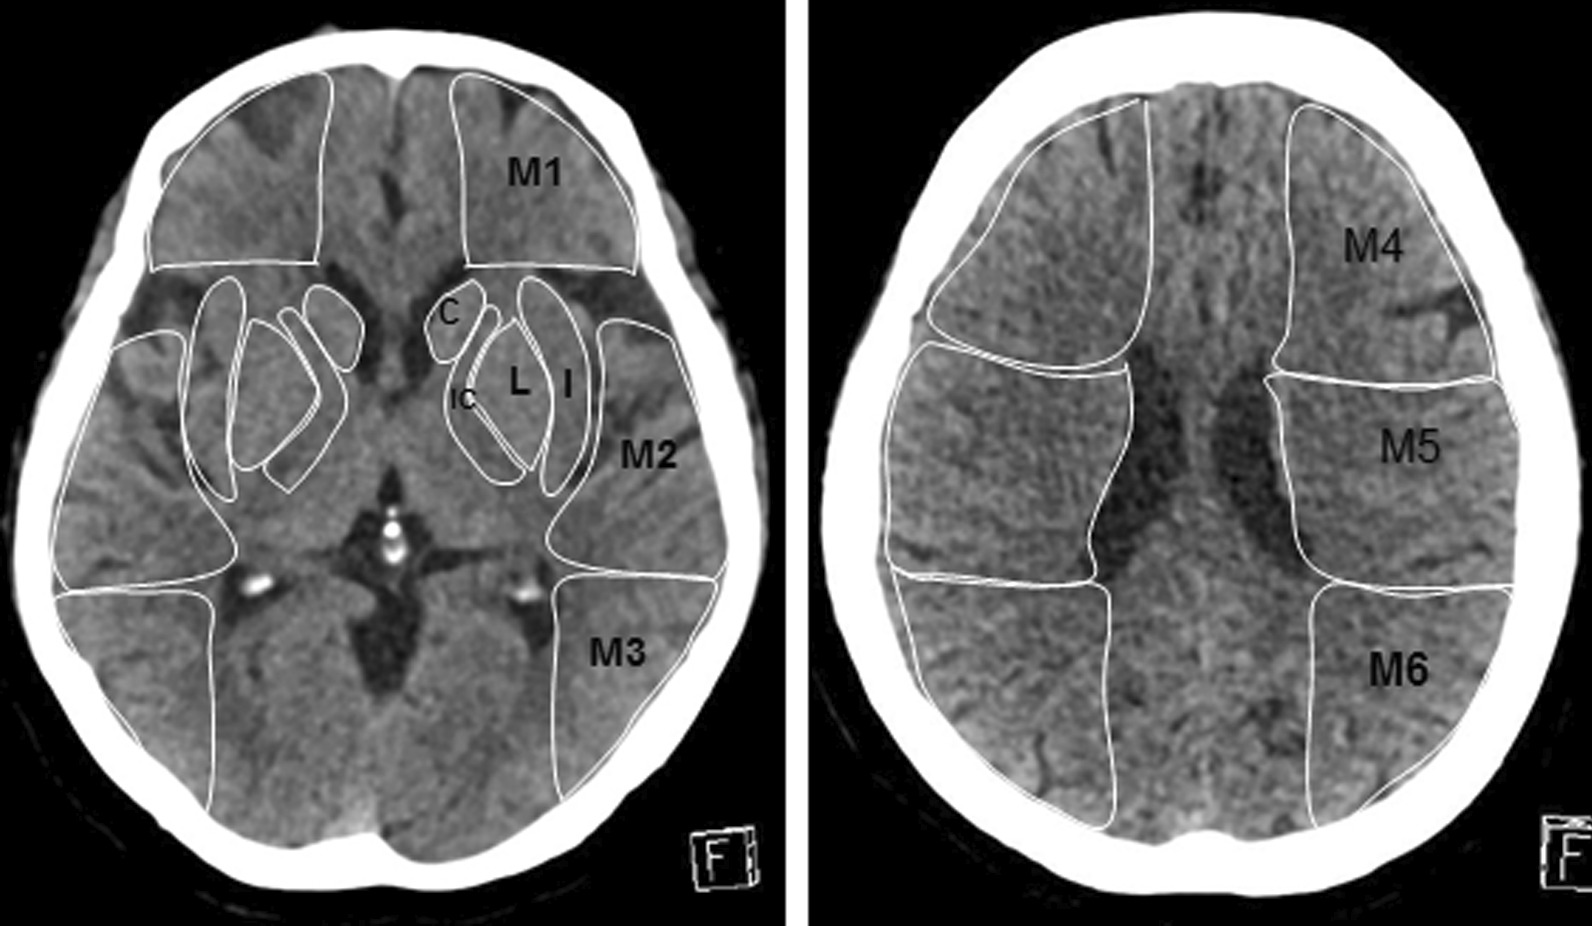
Ten ASPECTS regions were outlined on the NCCT images. HU ratio in ten ASPPECTS regions, C: 41.75/41.81, M1: 39.99/38.31, M4: 39.41/39.82, L: 41.82/41.29, M2: 42.11/44.54, M5: 37.26/37.72, IC: 31.67/34.03, M3: 41.19/38.85, M6: 38.95/39.04, I: 35.17/40.82. HU difference in ten ASPPECTS regions, C: |41.75–41.81|, M1: |39.99–38.31|, M4: |39.41–39.82|, L: |41.82–41.29|, M2: |42.11–44.54|, M5: |37.26–37.72|, IC: |31.67–34.03|, M3: |41.19–38.85|, M6: |38.95–39.04|, I: |35.17–40.82|
